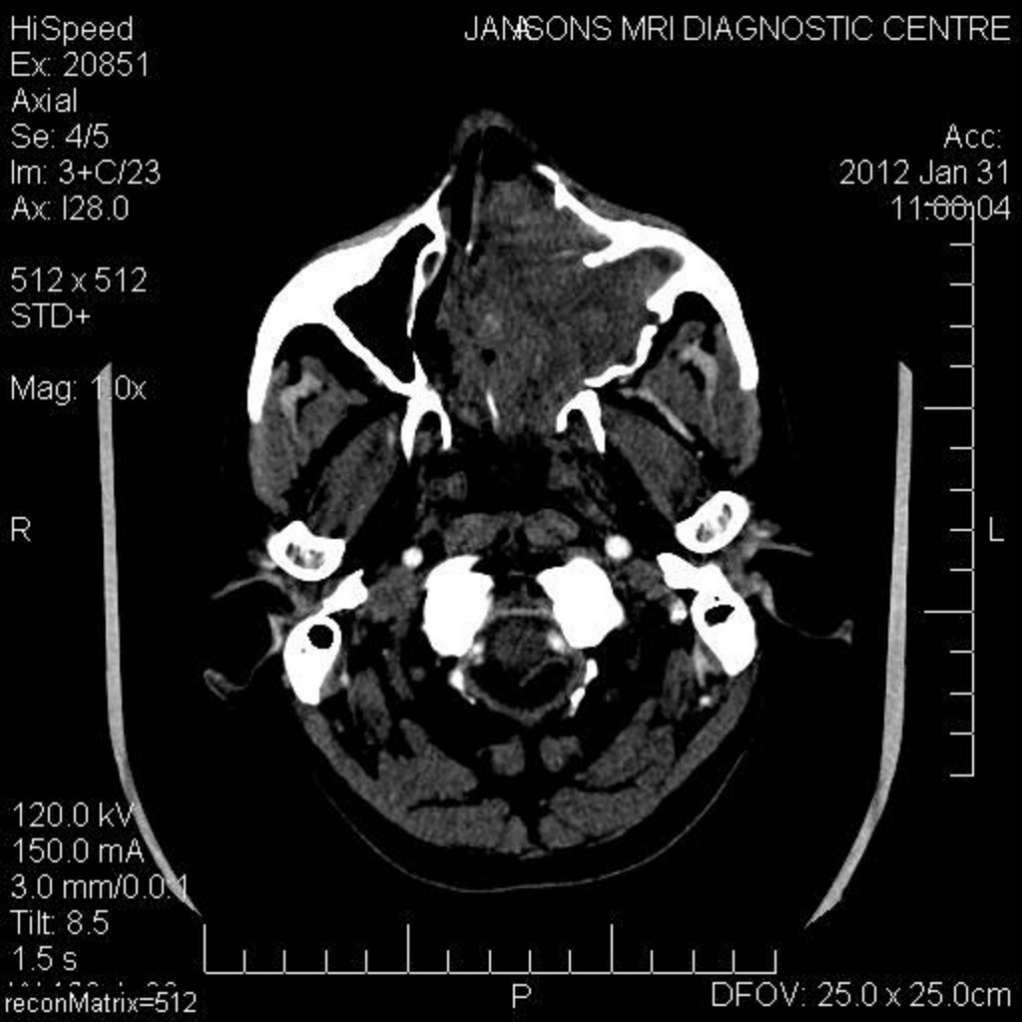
CT showing a large mass occupying the entire left nasal cavity and maxillary antrum, and also extending towards the right nasal cavity
